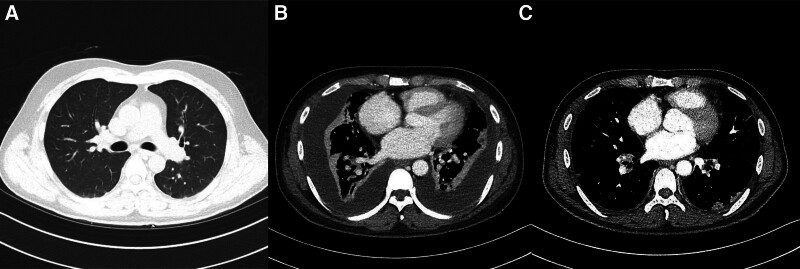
(A) Initial chest computed tomography without active lung lesions. (B) Chest computed tomography at the time of patient disorientation with bilateral pleural effusion and lung nodules. (C) Chest computed tomography after critical care.
